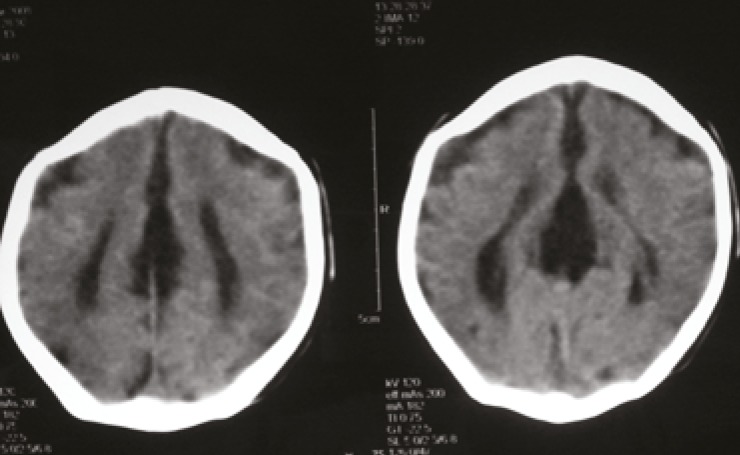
CT Brain, showing Agenesis of Corpus Callosum
